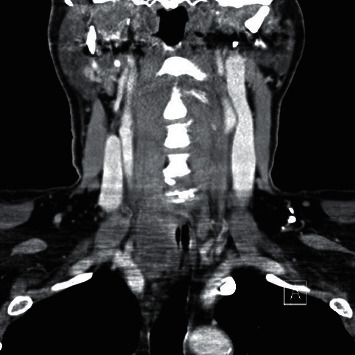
First CT scan of the neck after the initiation of pembrolizumab, 4 doses (coronal image).
